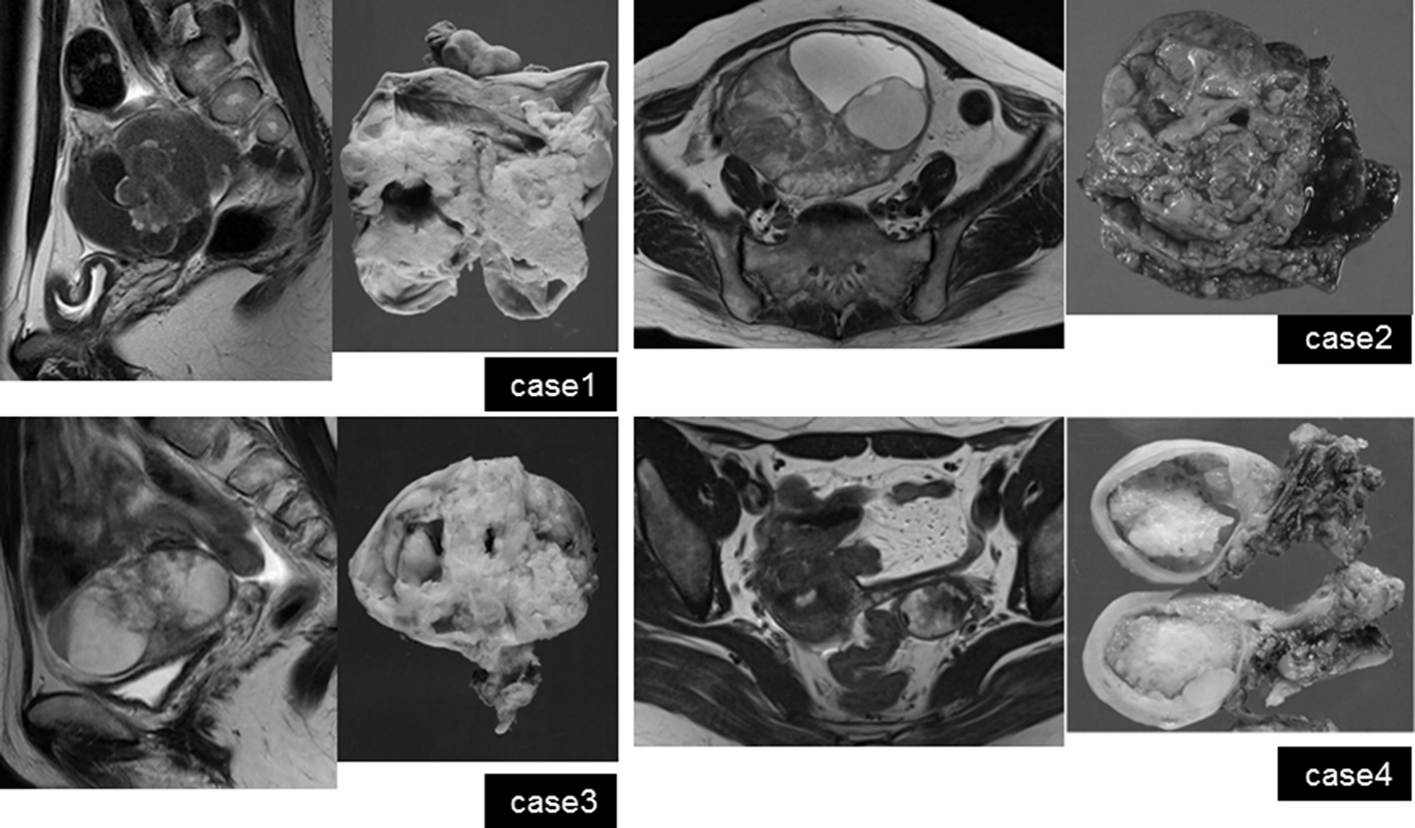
Imaging and macroscopic findings of case 1–4. *T*_2_WI (Cases 1–4) shows the tumours consisting of cystic and solid lesions. Reflecting abundant stroma, the components are of low signal intensity, and are of various shapes, including papillary projections on T2WI (Cases 2–4). Together with the high intensity on T_1_WI (data not shown), multicystic portions are assumed to have bloody content, but show variable intensity. The MR images are reminiscent of malignant tumours arising from endometriosis such as endometrioid carcinoma or clear cell carcinoma. T1WI, *T*_1_ weighted image; T2WI, *T*_2_ weighted image.
